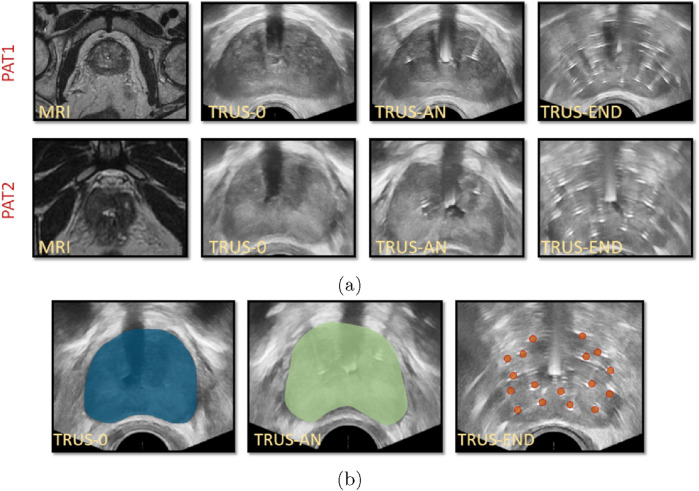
(A) Medical images for PAT1 and PAT2 including MRIs prior to catheter insertion, TRUS-0 (prior to catheter insertion), TRUS-AN (following the insertion of two anchor needles) and TRUS-END (ifollowing the insertion of all catheters). (B) Segmentation of TRUS-0 image, guided by the prostate MRI segmentation (*left*), segmentation of TRUS-AN (*middle*) and identification of catheters’ placement in TRUS-END, marked with red dots (*right*) for PAT2.
